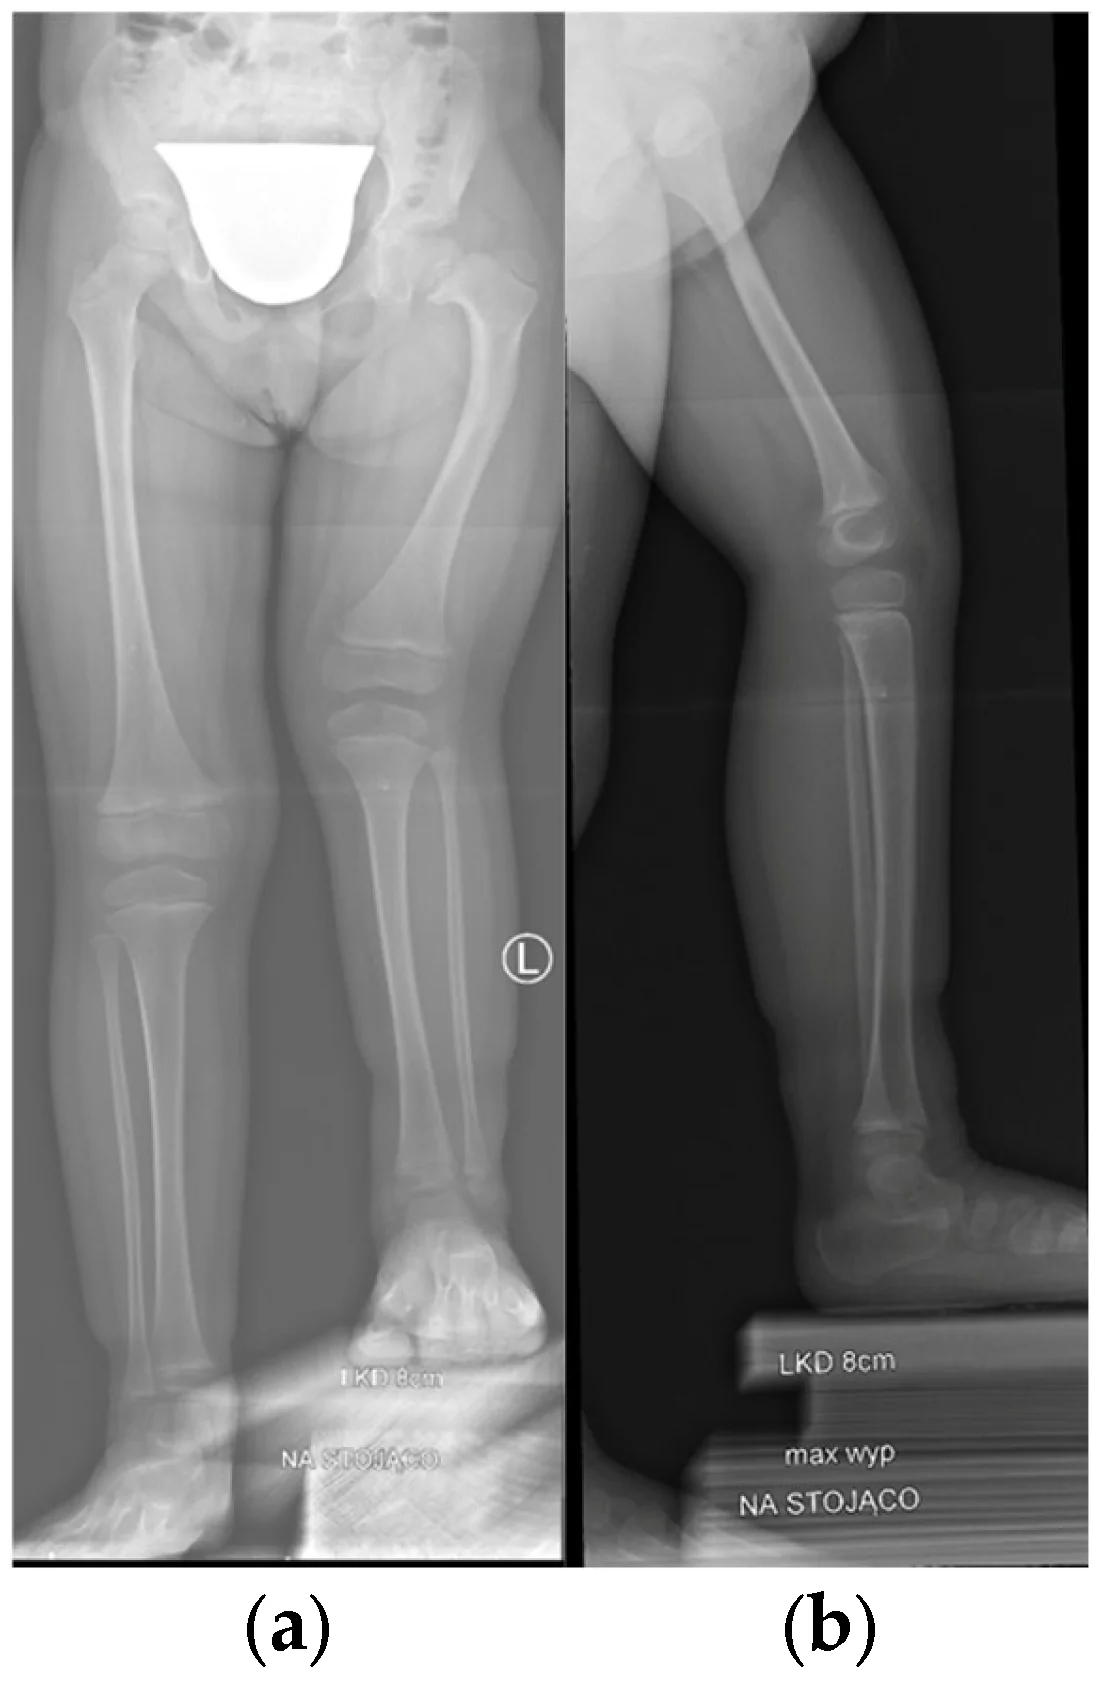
Standing radiographs of a 3.5-year-old patient with left-sided CFD type 1A3 and 9 cm shortening of the left lower limb: (a) anteroposterior; (b) lateral view. L—left, LKD—left lower limb, NA STOJĄCO—standing position, max wyp—complete knee extension.
